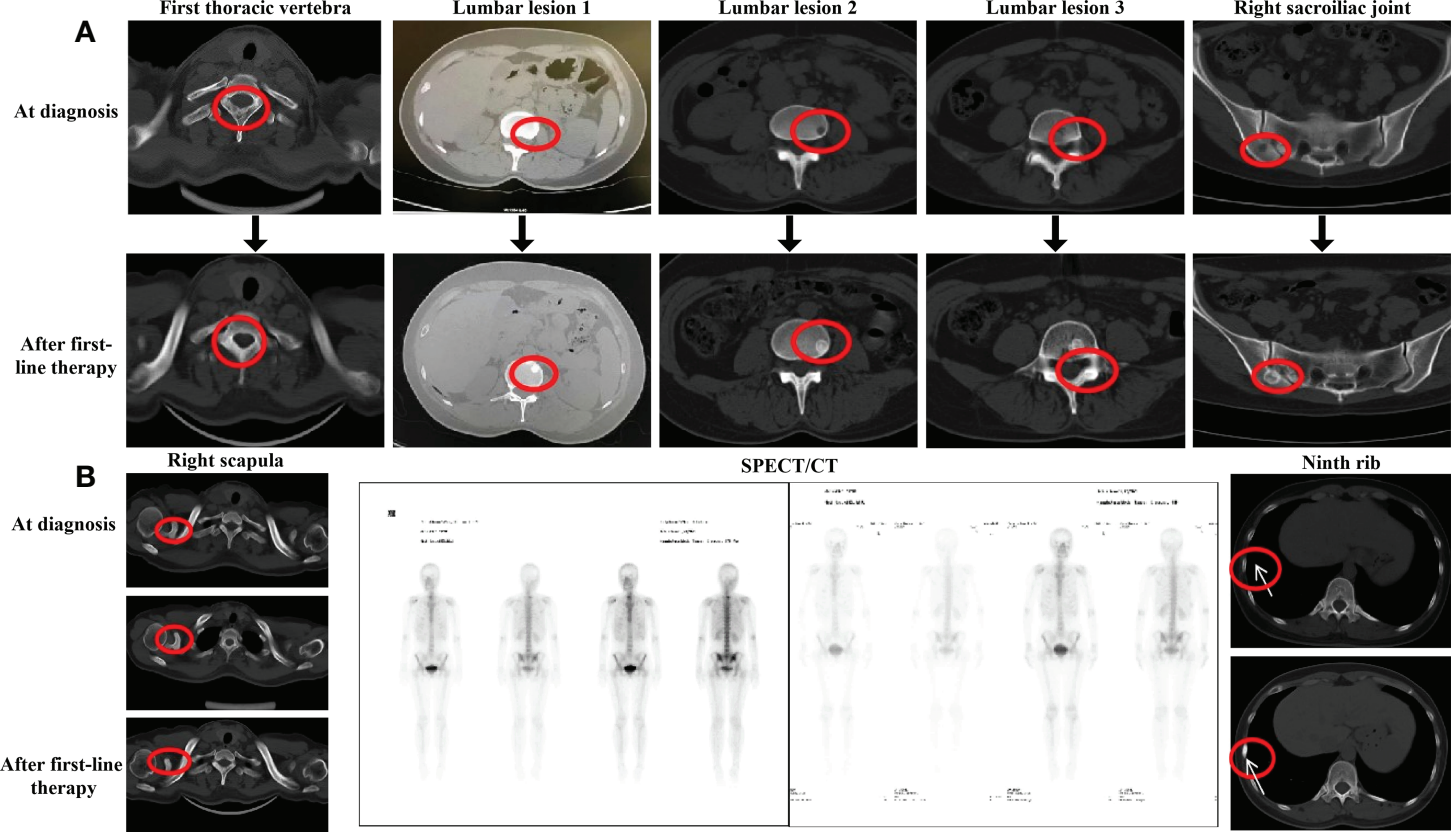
Evaluation after first-line endocrine therapy (A) CT evaluation of the bons lesion after first-line endocrine therapy in September 2020. (B) CT evaluation of right scapula the ninth rib and SPECT evaluation after first-line endocrine therapy in September 2020.
